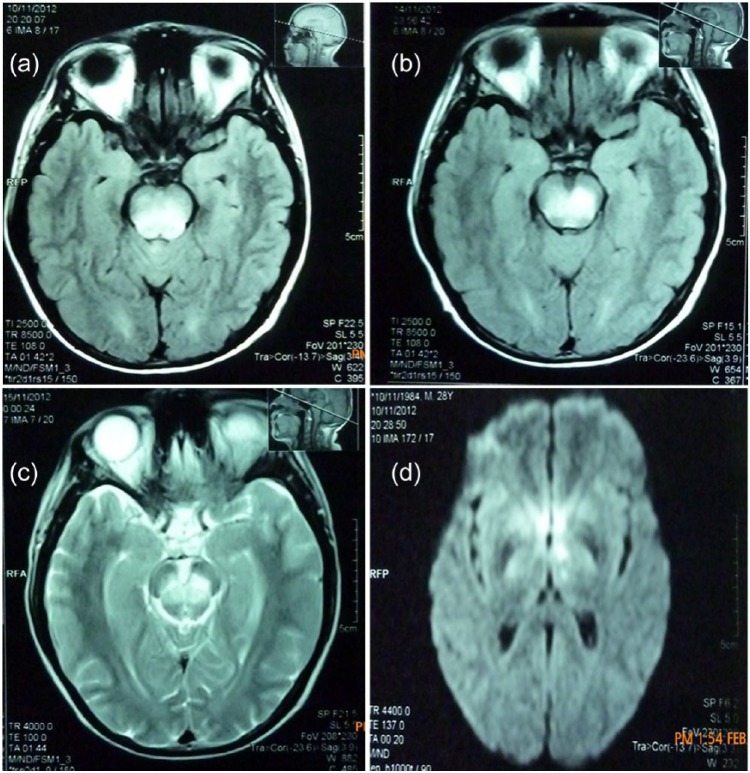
Acute IS within the pons on T1-weighted imaging (a), midbrain on T1- and T2-weighted imaging (b and c), and left thalamus on diffusion (d).
